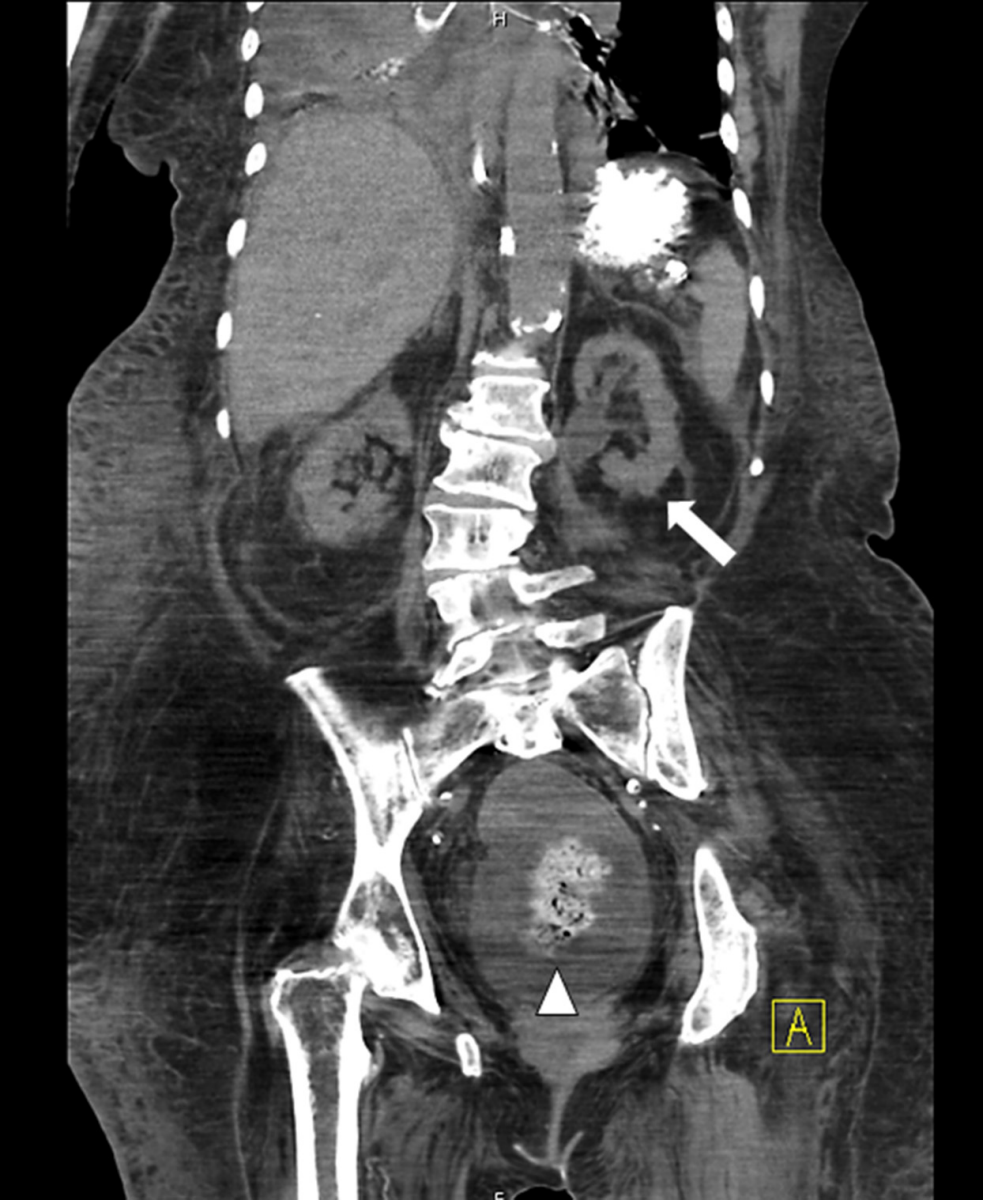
Noncontrast CT abdomen/pelvis, coronal view. Postdisimpaction and aggressive medical management. There is interval decrease in size of the rectal fecalith to 4 cm (white arrow) with persistent hydronephrosis and hydroureter (triangular arrow).
